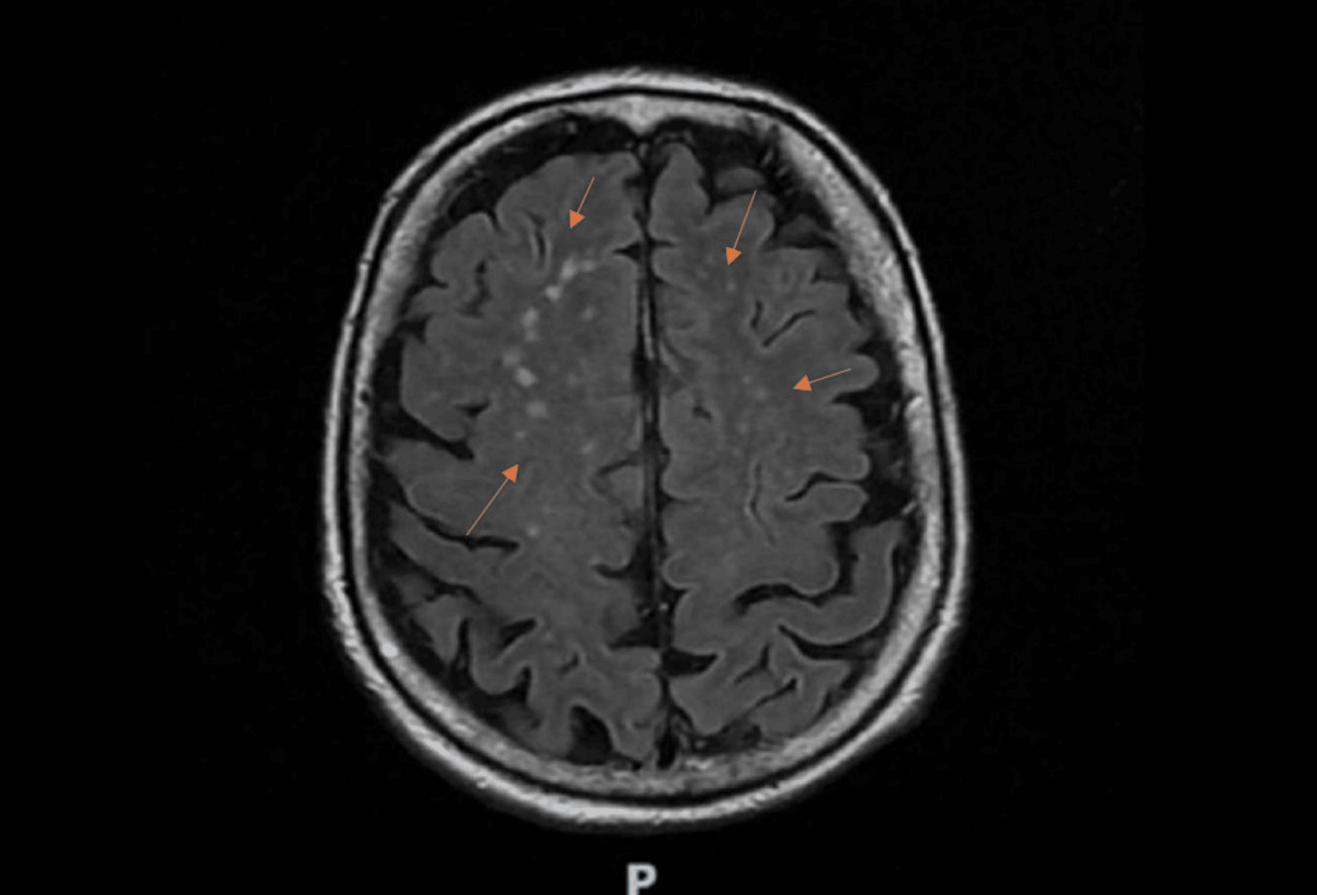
Axial T2 fluid-attenuated inversion recovery (FLAIR) magnetic resonance imaging (MRI) demonstrating faint diffuse cortical hyperintensity, more pronounced in the temporoparietal regions (arrows).
